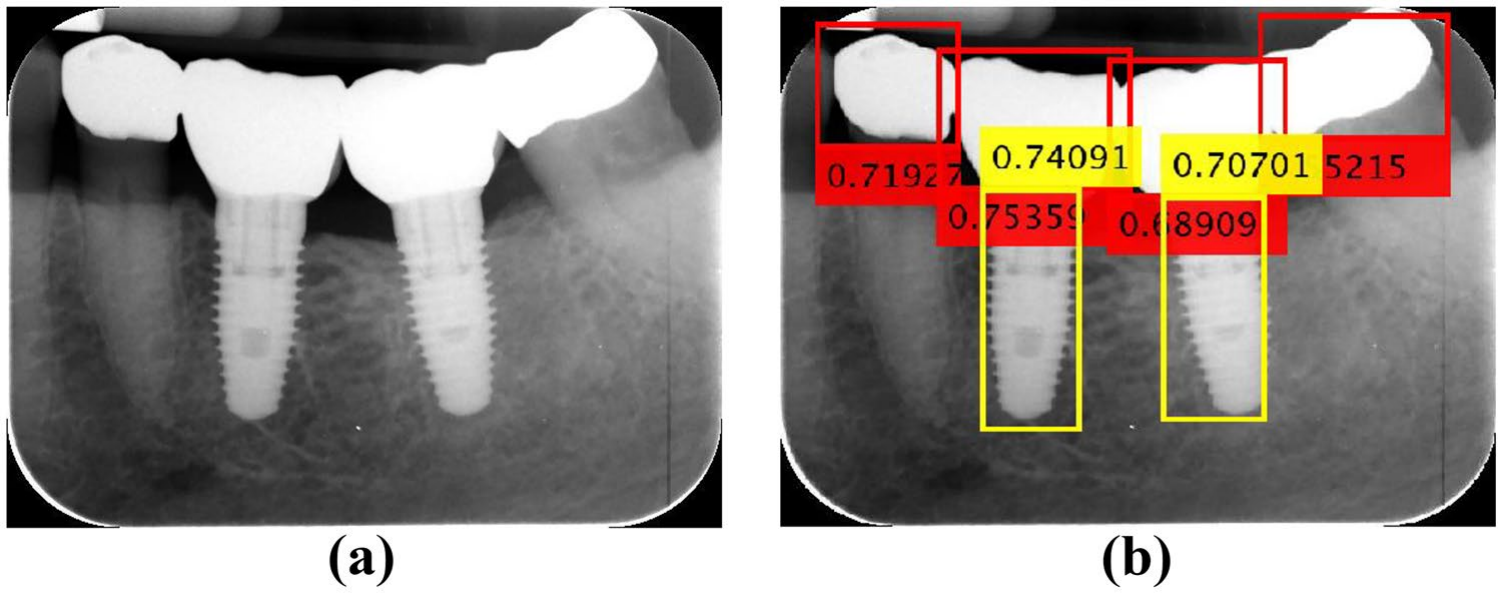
Periapical radiography: a original; b detected objects (crowns and screws)
